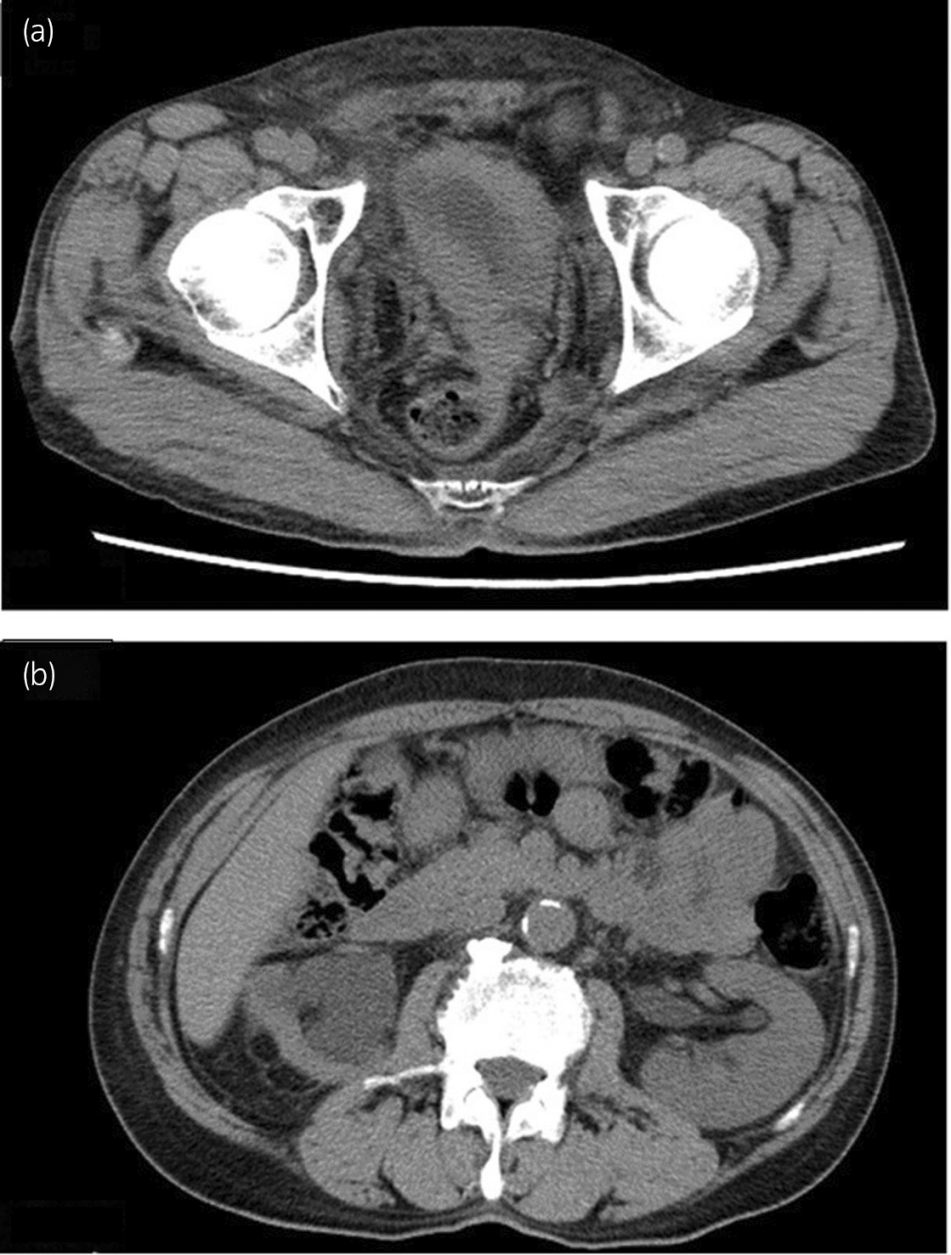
CT shows a thickened bladder wall and contiguous tumor extending into the rectum (a) as well as bilateral hydronephrosis and right renal atrophy (b).
